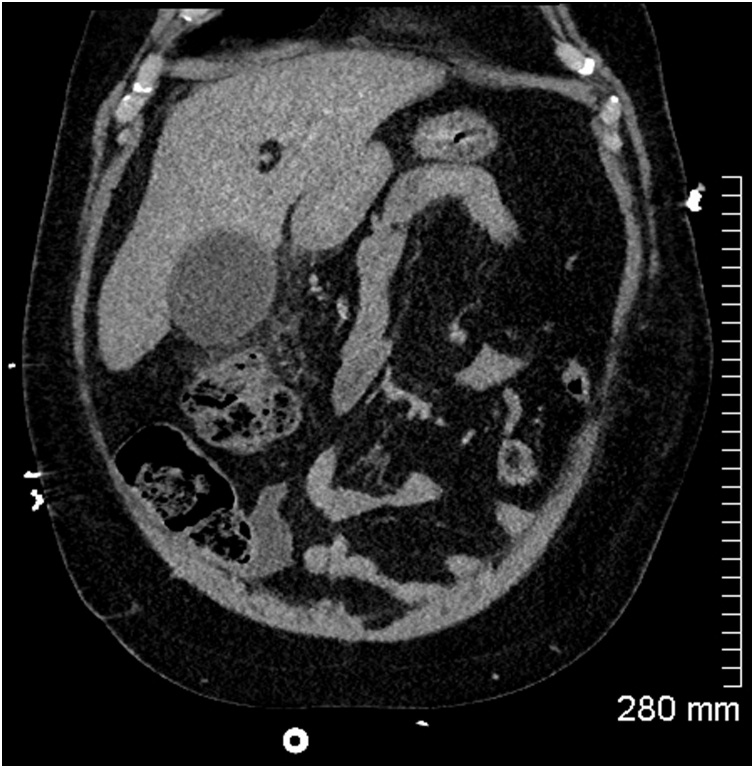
Coronal CT abdomen showing a distended gallbladder and pericholecystic fat stranding consistent with acute cholecystitis.
